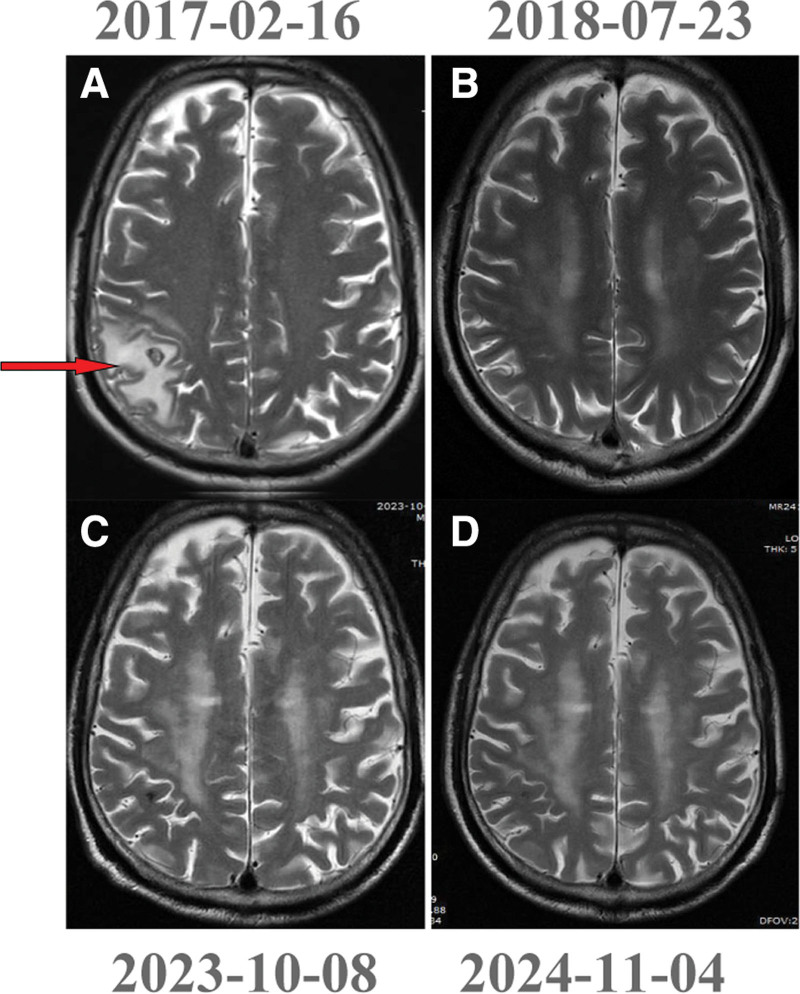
Brain MRI images. (A) Brain metastasis and edema after 3 postoperative cycles of paclitaxel plus cisplatin. (B–D) No recurrence after 16, 79, and 92 months of whole-brain radiotherapy combined with bevacizumab. MRI = magnetic resonance imaging.
